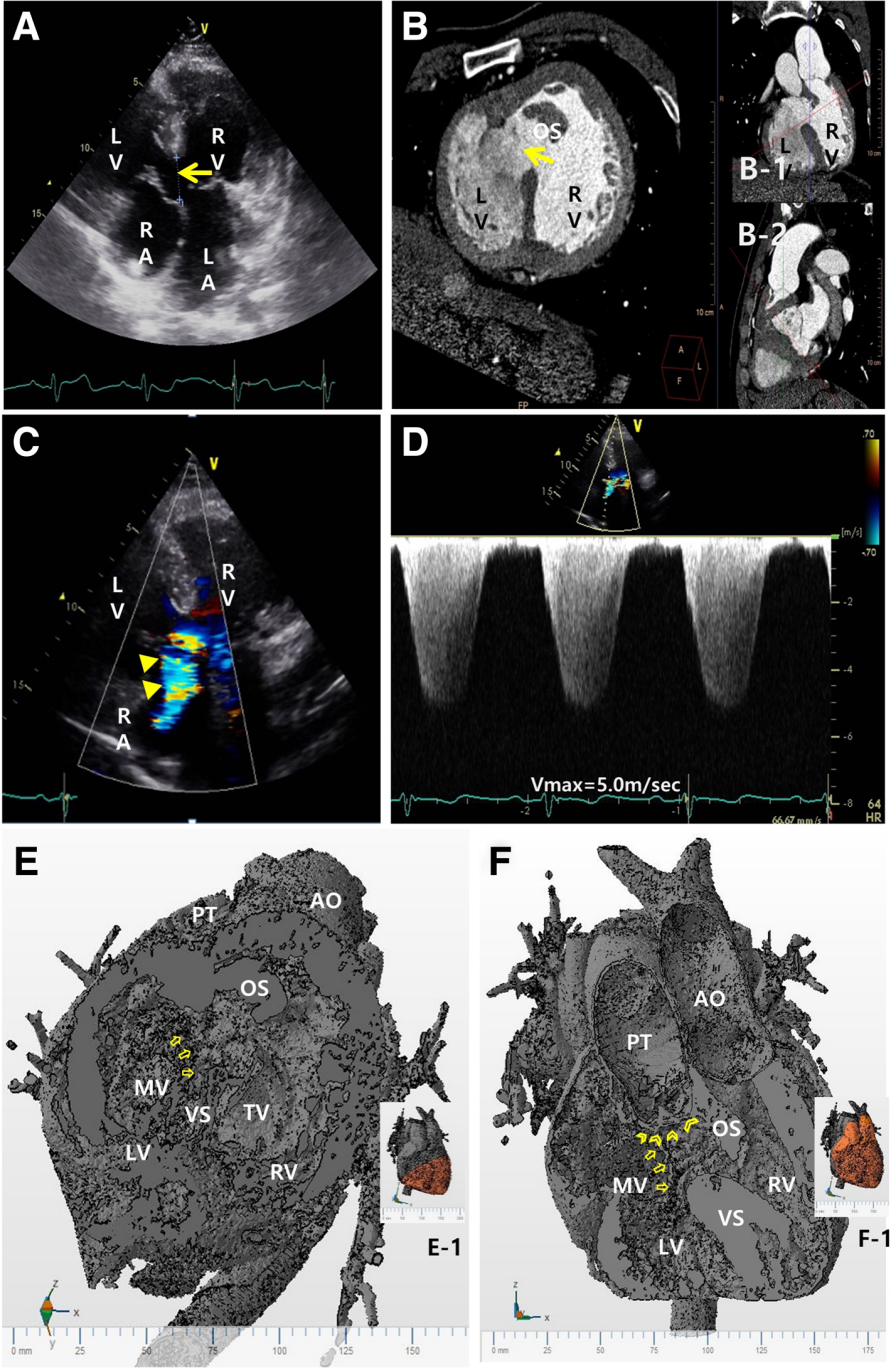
a Apical four-chamber view on transthoracic echocardiography shows a large ventricular septal defect (*arrow*). b Cardiac computed tomography images at the cutting planes shown in *red lines* in B-1 and B-2 reveal a large ventricular septal defect and prominent outlet septum. c and d Transthoracic echocardiography with color Doppler shows regurgitation of the right-sided atrioventricular valve (mitral valve) (*arrow heads*), with a peak velocity of 5.0 m/second. e A three-dimensional model of the cardiac computed tomography at a similar plane to that in (b) (as shown in E-1) is shown. The outflows to the aorta and pulmonary trunk are separated by a prominent outlet septum. The anterior mitral leaflet straddles the ventricular septum and is anchored to the ventricular septum (*arrows*). f A three-dimensional model of the cardiac computed tomography at a plane similar to that in Figs. 1B-1 and 3B-1 (as shown in F-1) is shown. The mitral leaflet (*arrows*) is anchored close to the pulmonary valve (*arrow heads*). There is a significant malalignment between the ventricular septum and outlet septum. (Supplementary video is available in Additional files 1 and 2). *AO* aorta, *LA* left atrium, *LV* left ventricle, *MV* mitral valve, *OS* outlet septum, *PT* pulmonic trunk, *RA* right atrium, *RV* right ventricle, *TV* tricuspid valve, *VS* ventricular septum
